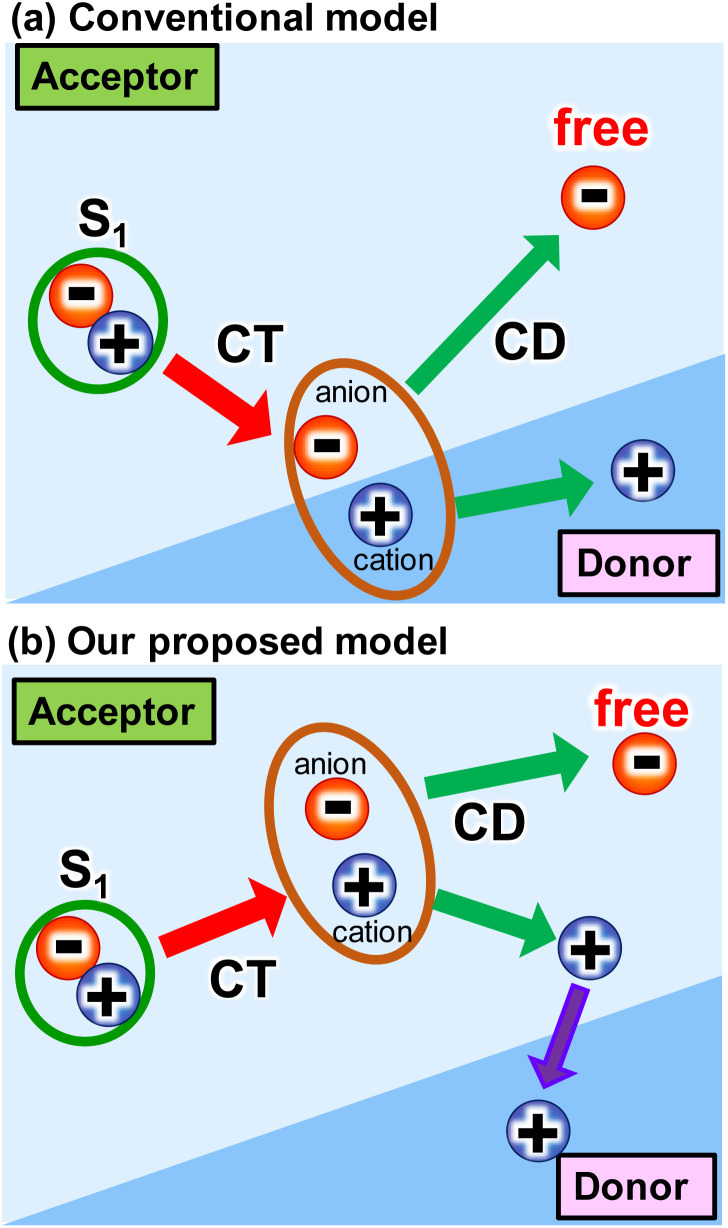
Several intermolecular charge transfer (CT) and charge dissociation (CD) models from singlet (S_1_) state in OPVs.
